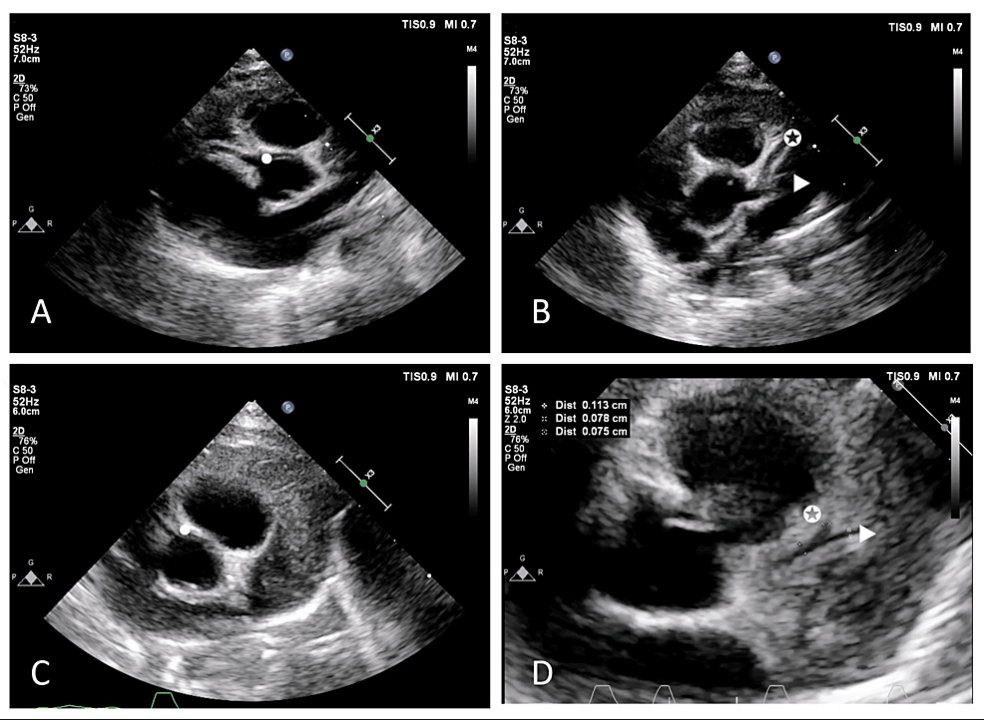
Echocardiogram (modified high parasternal short-axis view at the level of the aortic valve) A) and B) Presence of perivascular brightness with generalized dilatation and small aneurysm of RCA (dot), LAD (star), and LCx (triangle). C) and D) Follow-up echocardiogram showing regressed to normal size of all coronary arteries. LAD, Left anterior descending coronary artery; LCx, Left circumflex artery; RCA, Right coronary artery
